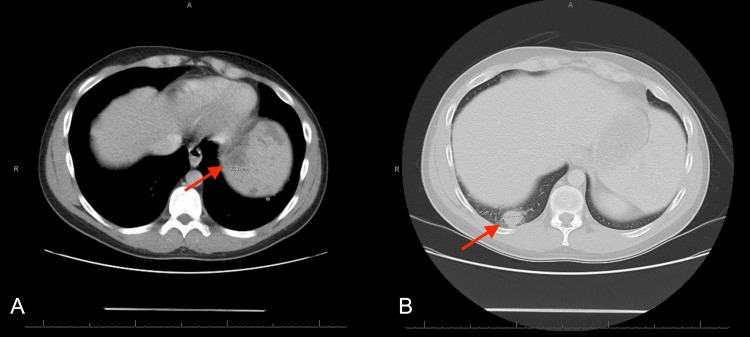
CT chest, abdomen, and pelvis with IV contrast (A) Multiple heterogeneous low attenuation lesions identified throughout the spleen, measuring up to 3 cm, significantly increased from prior CT. (B) Lung nodule in the right lower lobe measuring up to 2.5 cm increased from prior CT.
